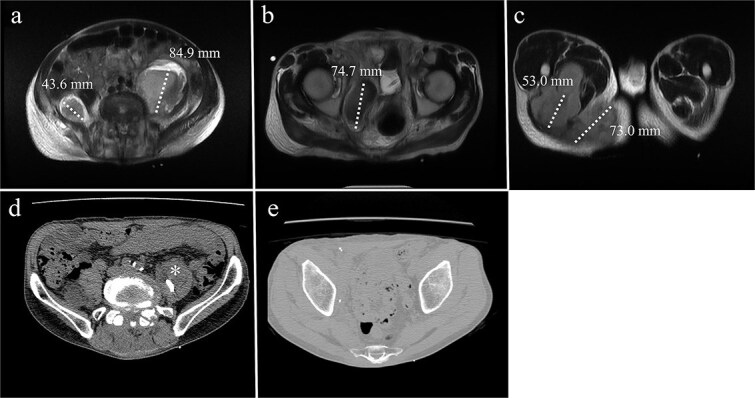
MRI of a 68-year-old male with (a) bilateral psoas abscesses (right: 43.6 mm, left: 84.9 mm). The right abscess extended caudally to (b) the quadrilateral plate of the acetabulum and (c) the medial thigh and ischial tuberosity region. (d) Partial resolution of the right abscess with persistence of the left abscess (*). (e) Complete resolution of the right caudal extension after debridement.
